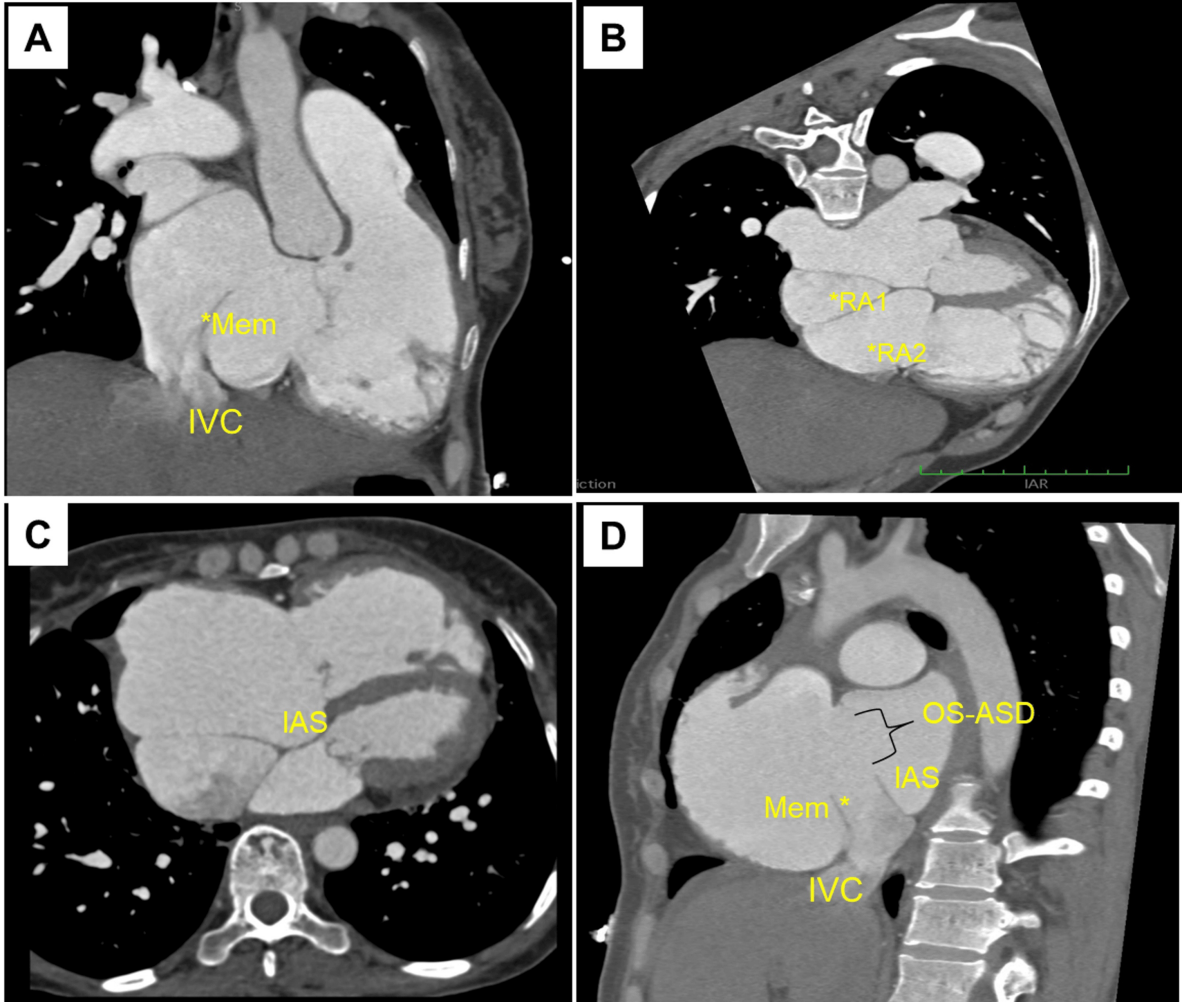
Cardiac computed tomography images. (A) Right ventricle two-chamber view showing a membrane from the anterior rim of inferior vena cava (IVC). (B, C) Four-chamber view showing a divided right atrium (RA1 and RA2) due to a membrane, along with a large ostium secundum atrial septal defect (OS-ASD). (D) Modified biatrial view showing the membrane (mem), IVC, interatrial septum (IAS) and OS-ASD.
